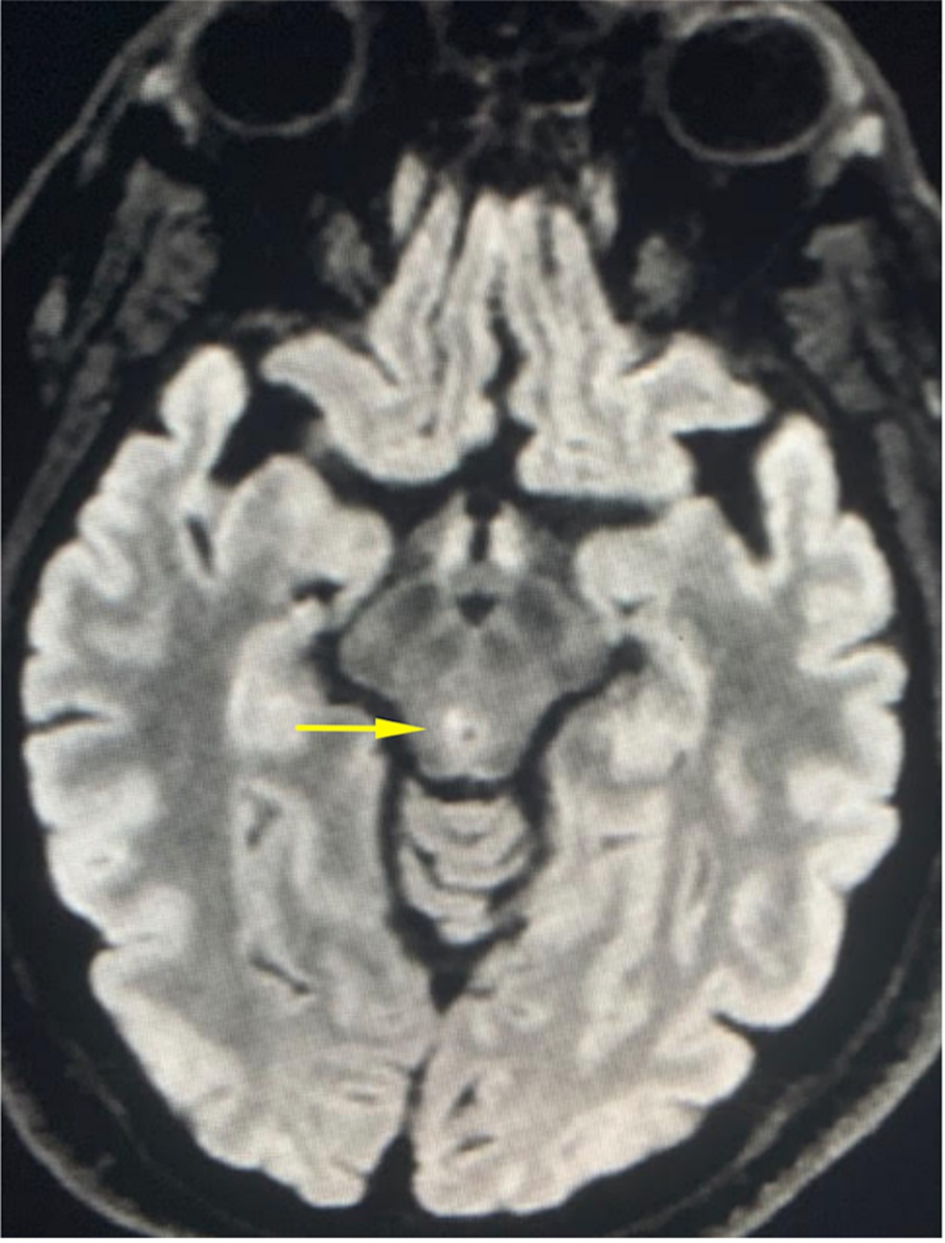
Axial FLAIR demonstrating oculomotor nuclear lesion FLAIR: fluid-attenuated inversion recovery
